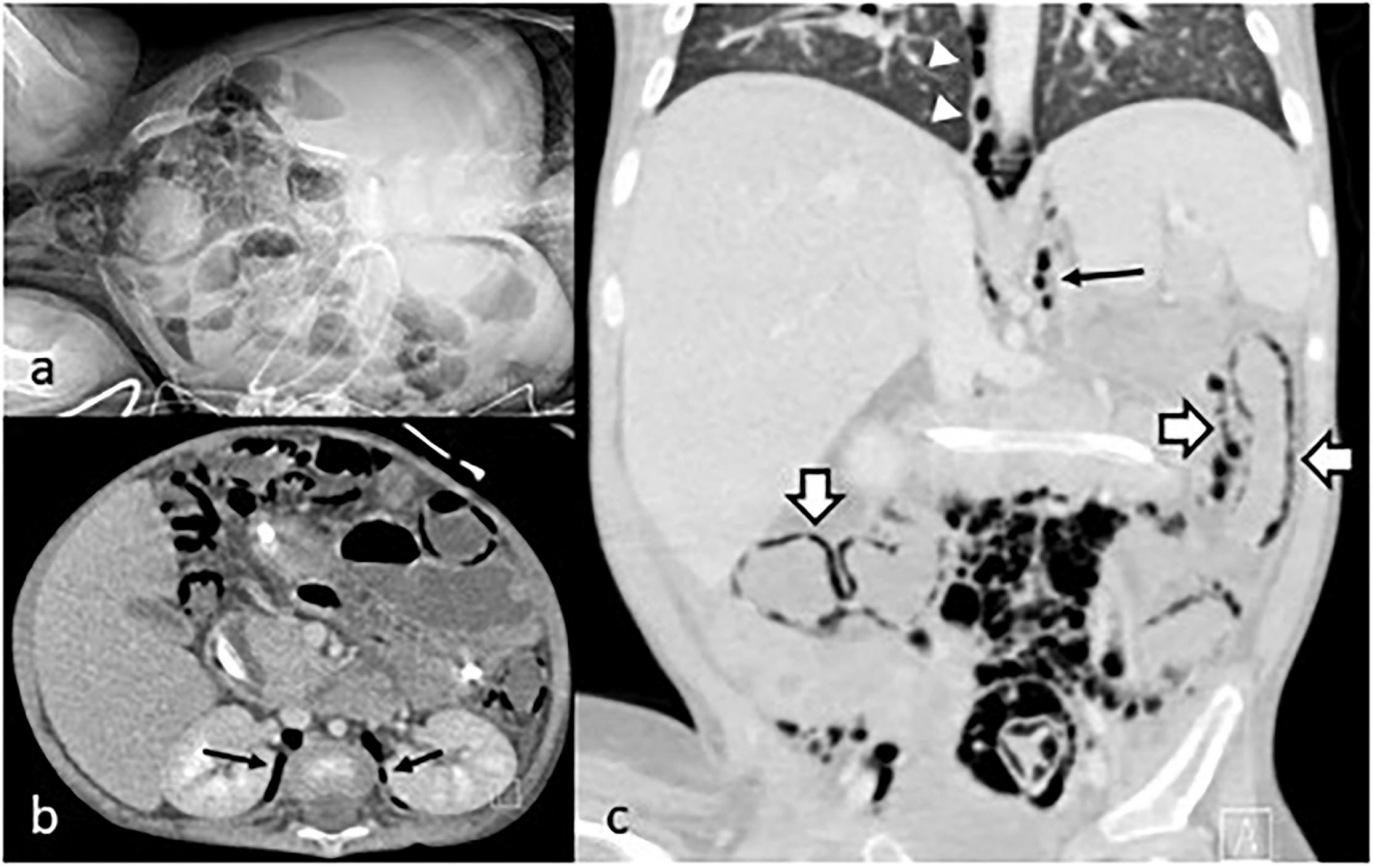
(a) Abdominal x-rays showing IP; (b) coronal CT scan with PRP (arrows); (c) sagittal CT scan showing pneumomediastinum (arrowhead), PRP (arrow), and IP (empty arrows).
